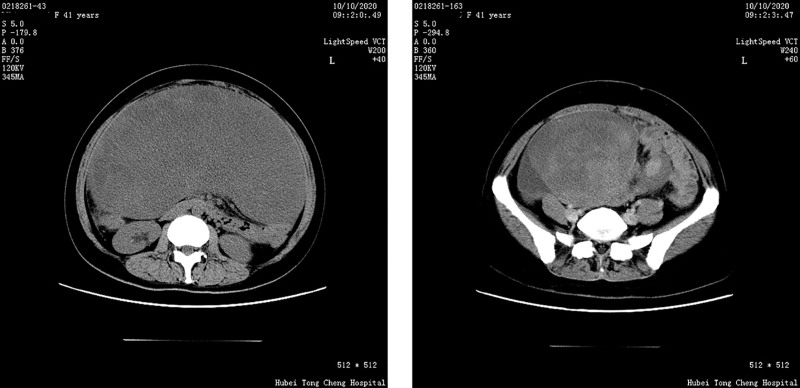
Preoperative CT image of patient in Case 1. CT = computed tomography.
